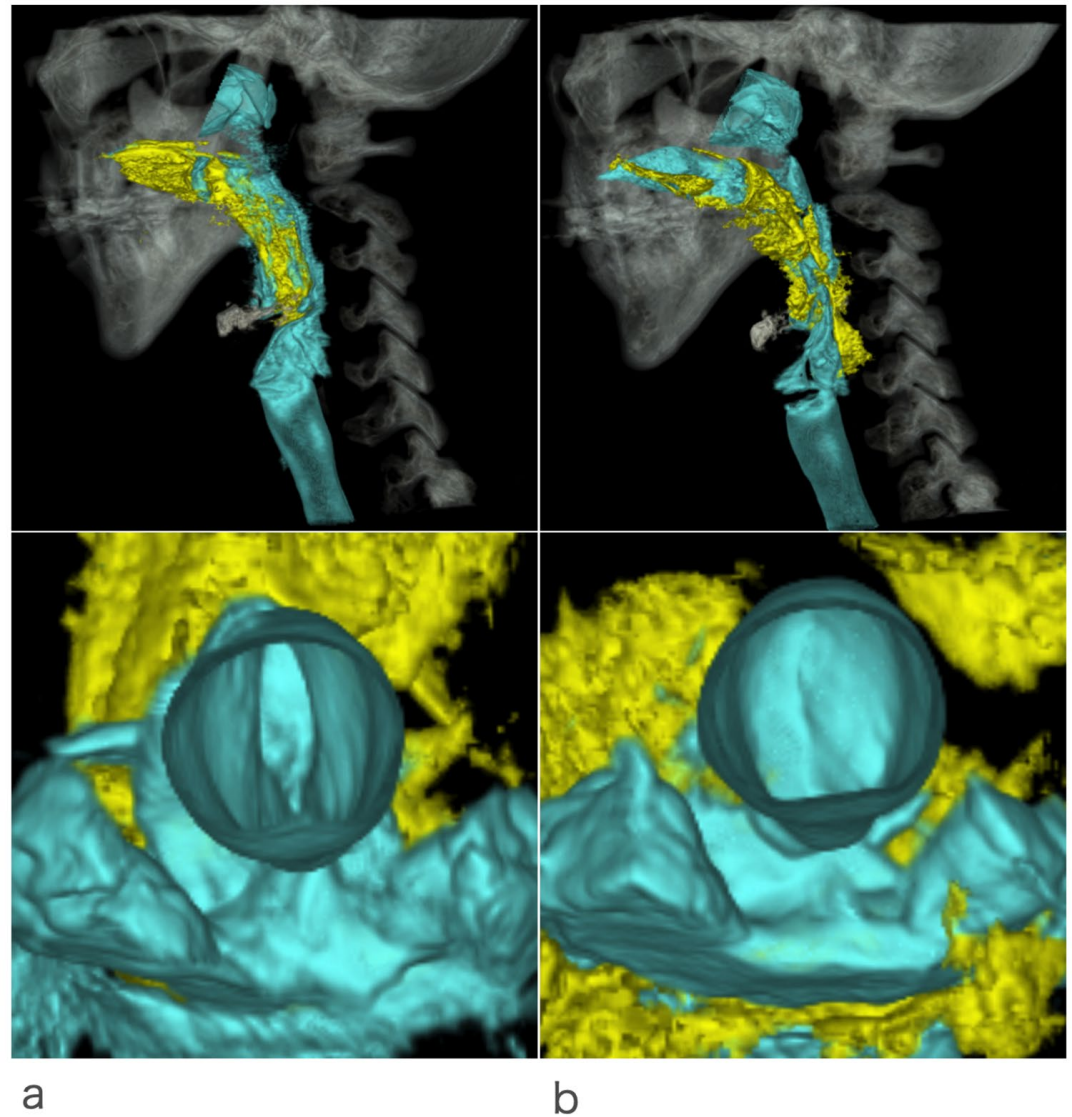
3D-CT images of one healthy volunteer’s swallow of honey thick liquid and thin liquid. Lateral image (upper) and transverse image (view of true vocal cord from below) (lower) at the onset of hyoid anterosuperior movement during honey thick liquid (a) and thin liquid (b). At the onset of hyoid anterosuperior movement, honey thick bolus was transported to valleculaetrue and vocal cord (TVC) was still open. Meanwhile, thin liquid bolus was transported to hypopharynx, lower than that of honey thick, and TVC was closed already
